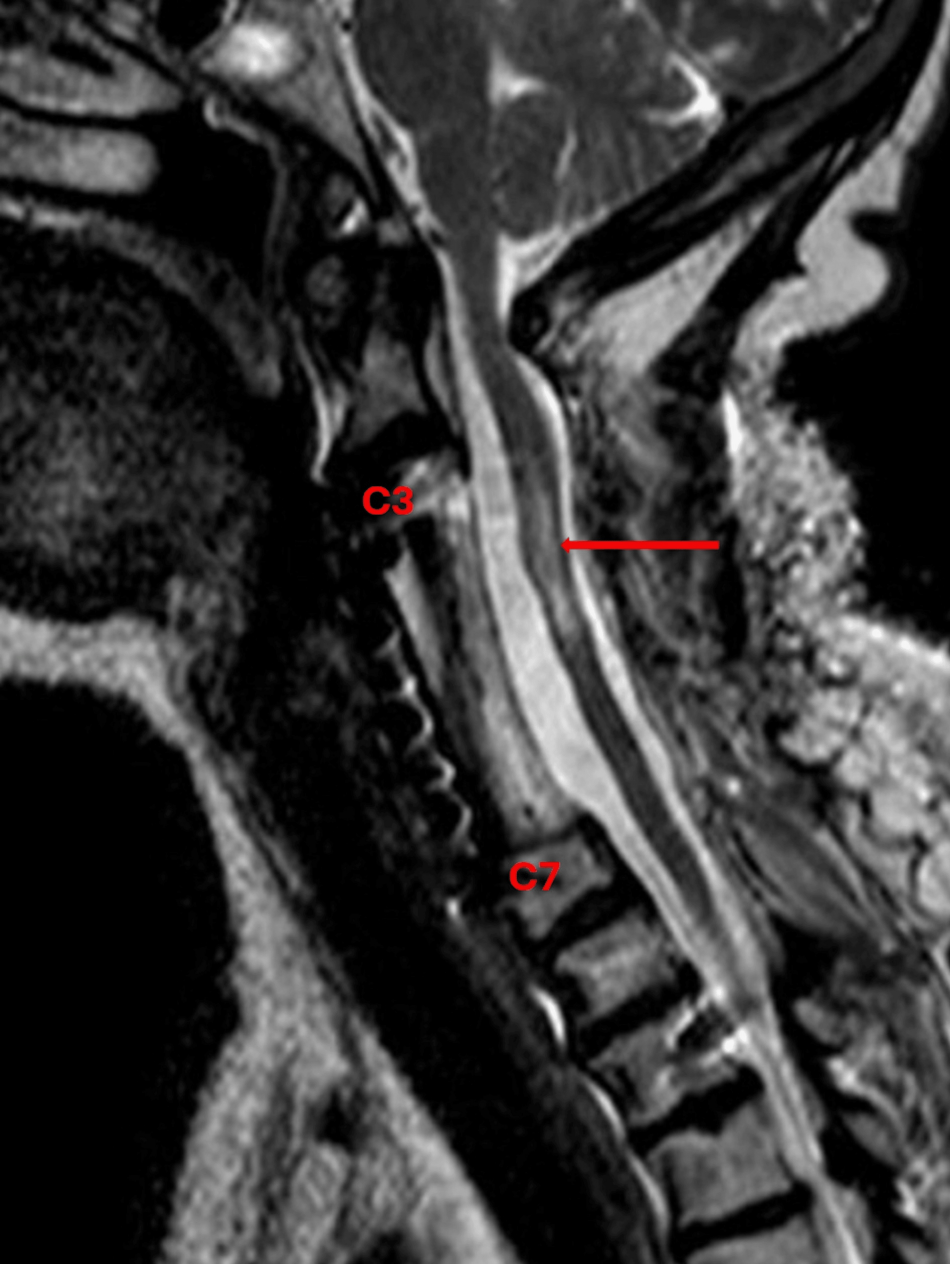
Postoperative cervical spine sagittal T2-weighted MRI. Red arrow shows a hyperintense signal at C4-C5 and surrounding spinal edema.
